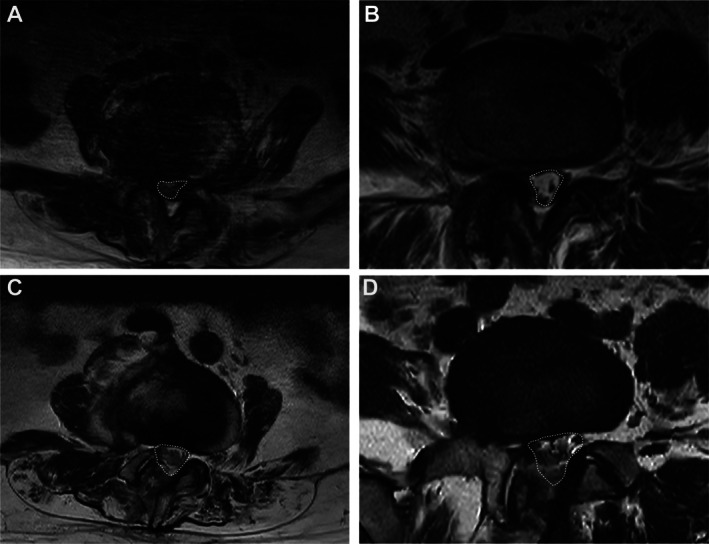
Preoperative and 1‐month postoperative axial T2‐weighted MRI at L4–5 showing DCSA changes. A, C: Non‐navigation (NON‐NAV), preoperative (A) and postoperative (C). (B, D) Navigation (NAV), preoperative (B) and postoperative (D).
